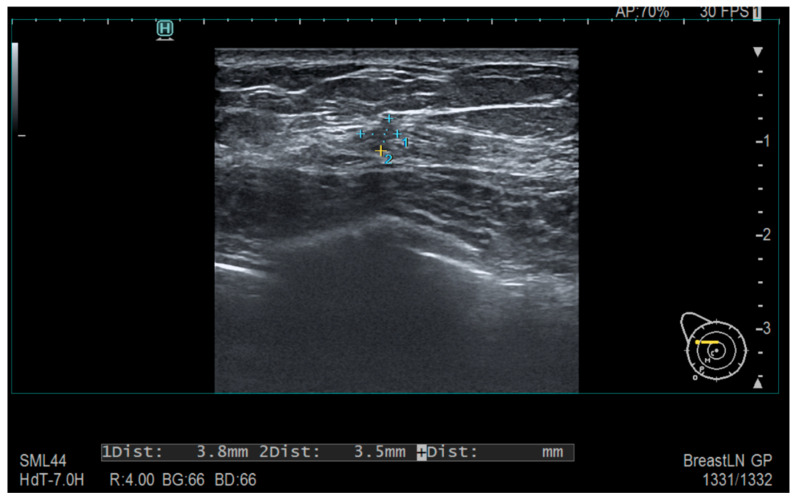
Ultrasonography—Diffusely contoured, hypoechoic area located in the external quadrant, measuring 3.8 mm × 3.5 mm.
